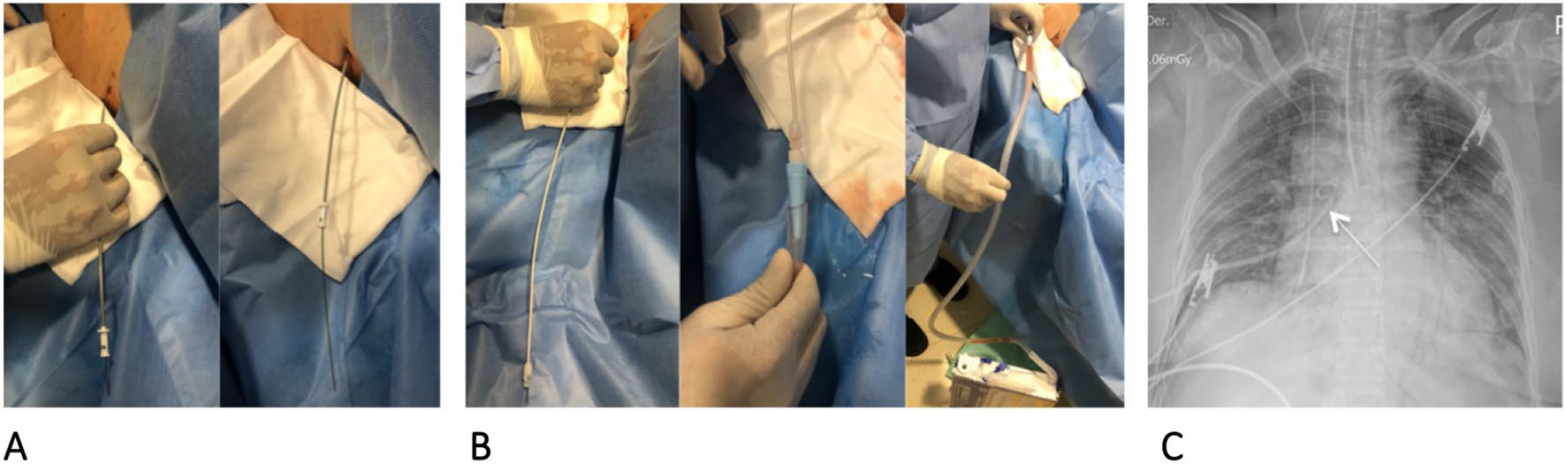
A Passage of the dilator, between 1 and 2 cm, depending on the thickness of the chest wall B. Passage of the pigtail catheter and connection to a thoracic drainage system C. Chest X-ray with complete lung re-expansion and pigtail in position (arrow)
